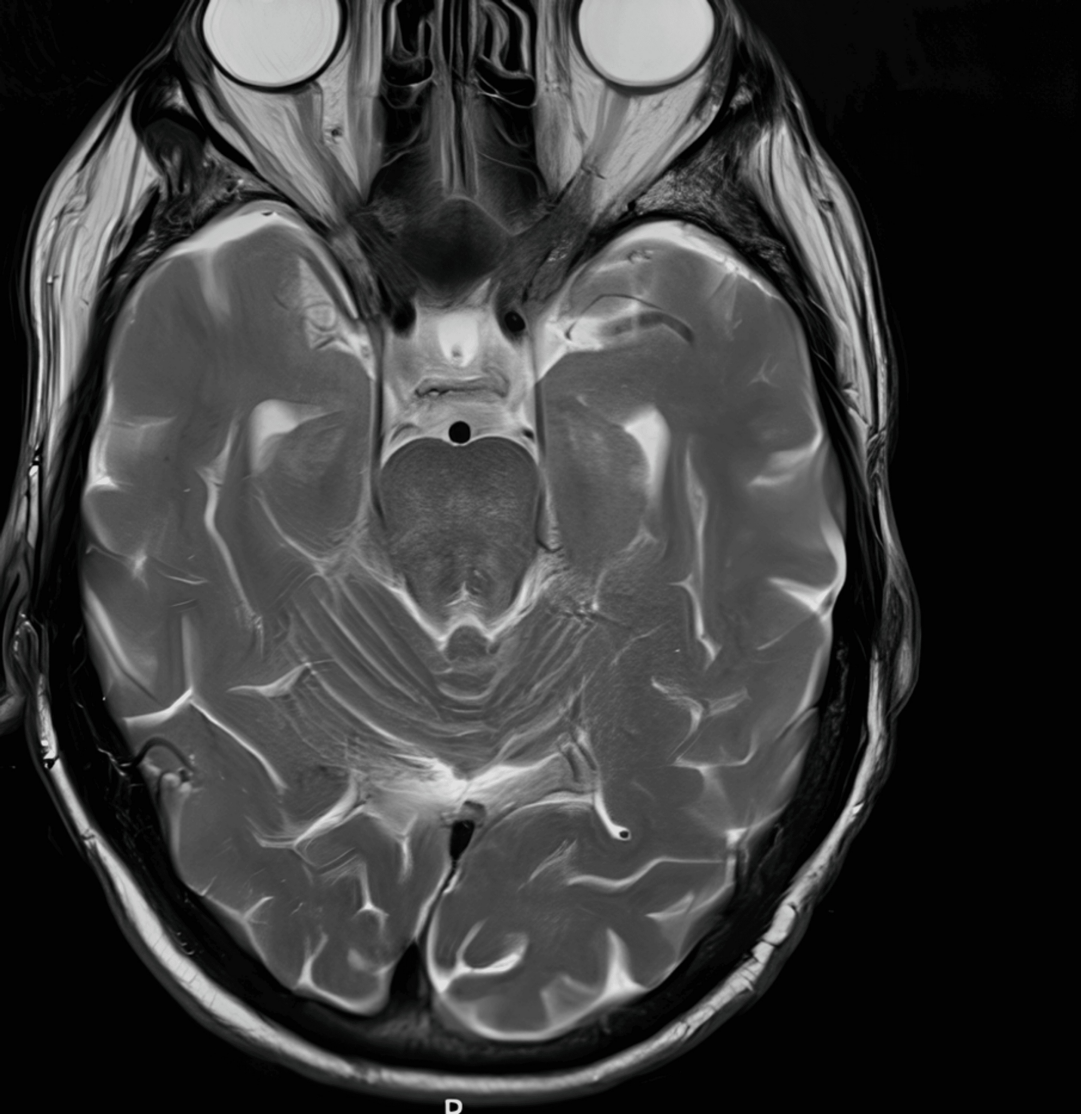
Brain MRI-axial section Axial T2-weighted MRI of the brain shows normal findings.
